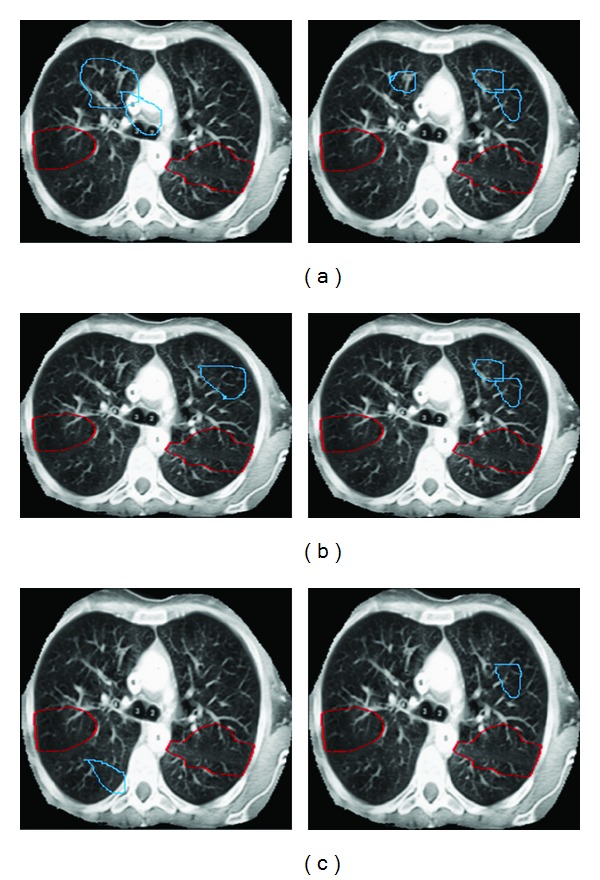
Comparison of performance based on different CT images (a) GICOV (b) circularity (c) hybrid.
